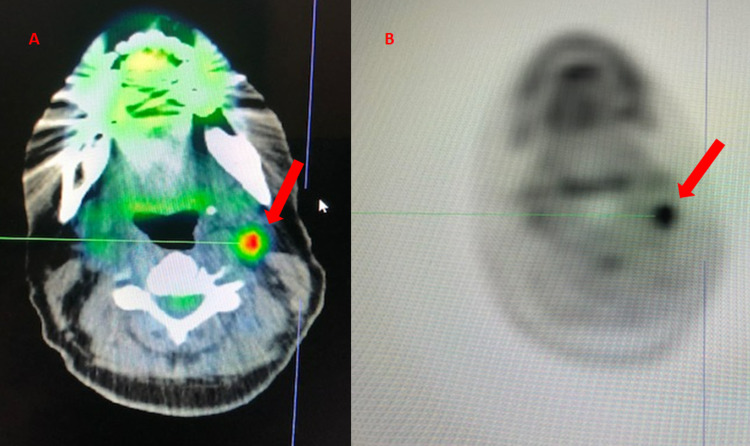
PET-CT one month after completion of ASCT. The images show (A and B) PET-CT completed one month after completion of ASCT demonstrating 18-FDG hypermetabolic uptake in level 2-3 left cervical lymph node less than 1 cm in size, SUV max 6.9. PET: positron emission tomography; ASCT: autologous stem cell transplant; FDG: fluorodeoxyglucose; SUV: standardized uptake value
